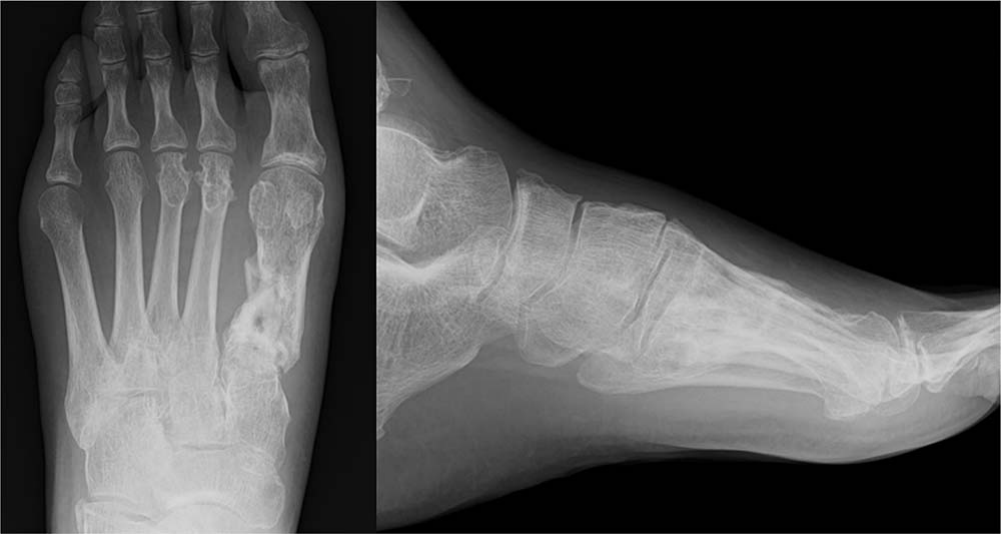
Radiography images 1 year after surgery.
